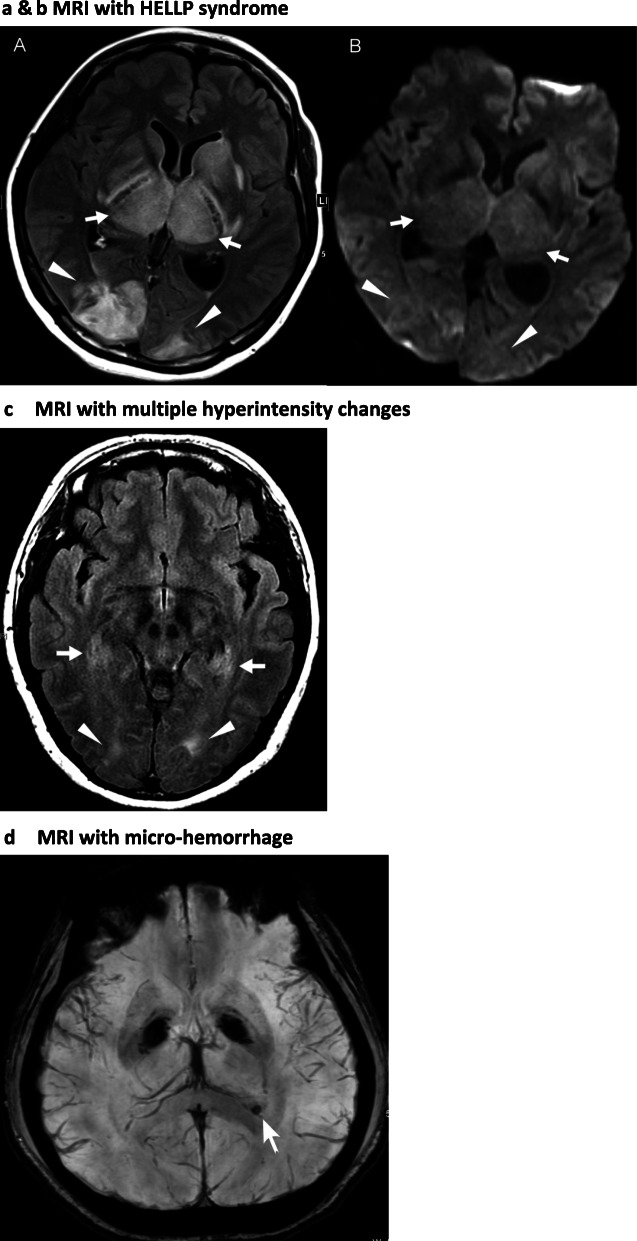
a & b MRI of patient with HELLP syndrome A 35-year-old pregnant women at 37 weeks of gestational experiencing severe pre-eclapmsia, headache and HELLP syndrome. Brain MRI revealing grossly symmetric hyperintensities over bilateral basal ganglia, thalami (white arrows) and occipital lobes (white arrowheads) on FLAIR, but minimal hyperintensities on DWI (b), consistent with typical PRES with vasogenic edema 2 (c) MRI with multiple hyperintensity changes Brain MRI of a 39-year-old pre-eclamptic pregnant women having headache at 28 weeks of gestation. Axial FLAIR disclosed symmetric hyperintensities on bilateral medial temporal lobes (white arrows) and occipital subcortical white matters (white arrowheads), suggestive of the mild form of PRES 2 (d) MRI with micro-hemorrhage A 25-year-old pregnant woman with gestational age of 26 weeks presented with headache and hypertension up to 200/100 mmHg. A profound hypointense focus on the periventricular white matter of left occipital horn was observed by SWI, indicating of a microhemorrhage (white arrow) and a precedent change of PRES onset
